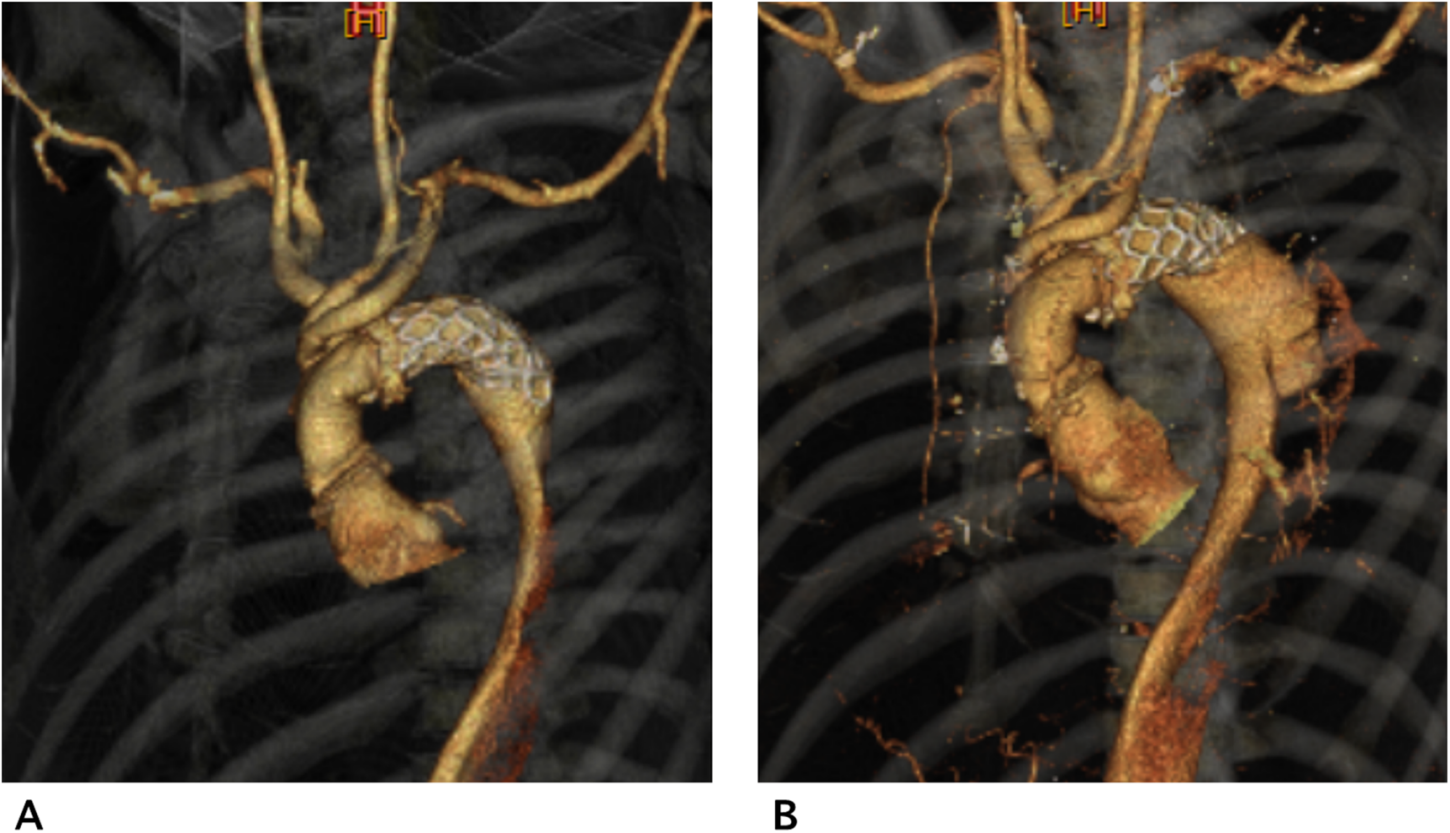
Computed tomography angiography (CTA) after type A aortic dissection repair showed poor remodelling with a narrow true lumen (TL) (A) CTA after dSINE, which occurred 2 months later after TAAD repair showed aortic enlargement and a residual narrowed TL (B).
